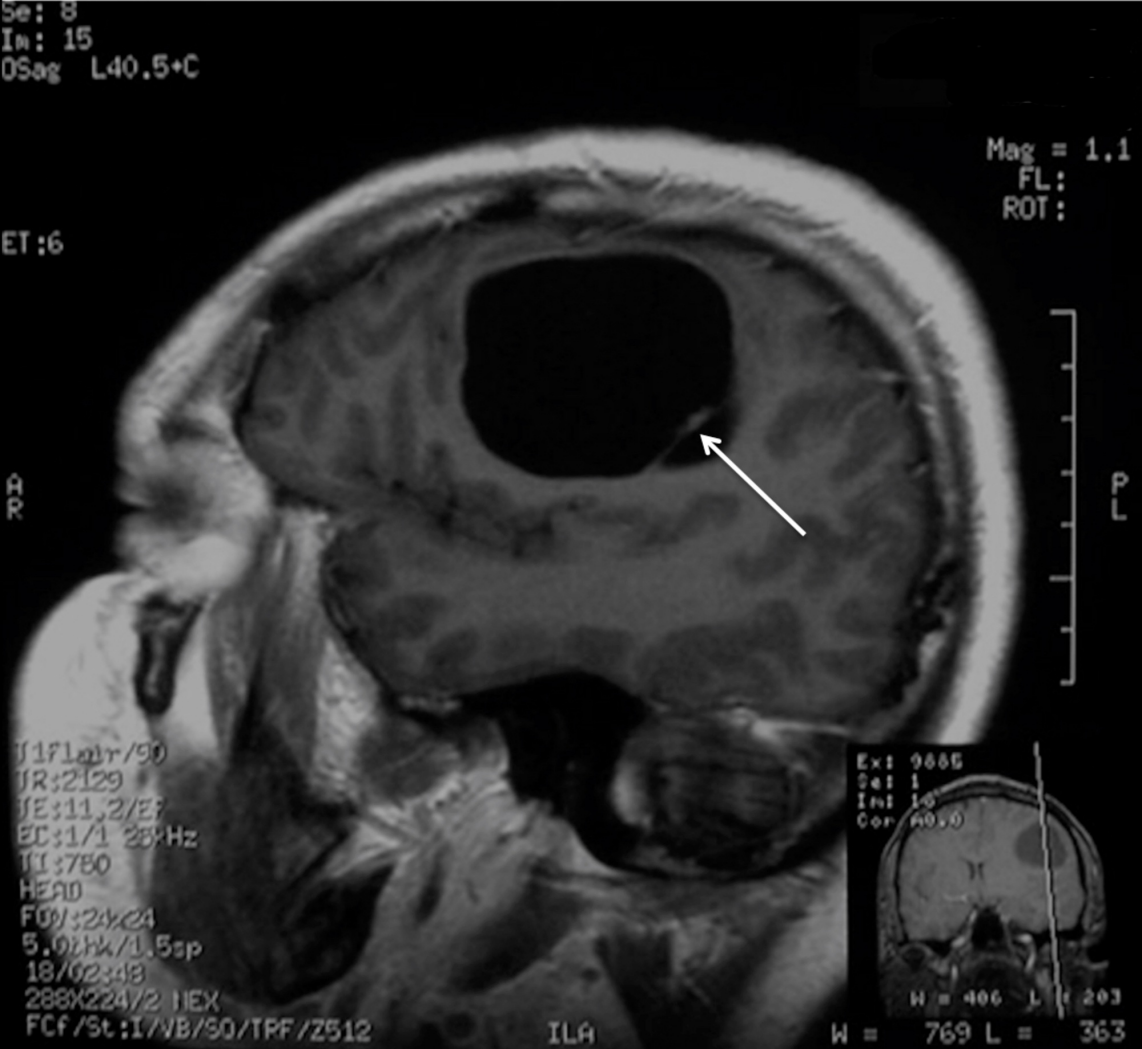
Preoperative MRI demonstrating the contrast-enhancing septum (arrow) in the posterior part of the cyst on the T1WI sagittal view. MRI: Magnetic resonance imaging; T1WI: T1-weighted images.
